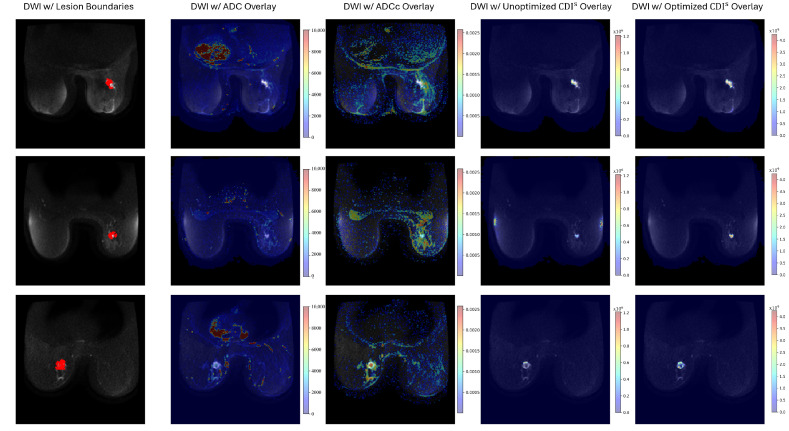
DWI images with overlays of the lesion boundaries, ADC, ADCc, Unoptimized CDI^s^, and Optimized CDI^s^ where the red pixels refer to the lesion for the specific DWI on the far left.
